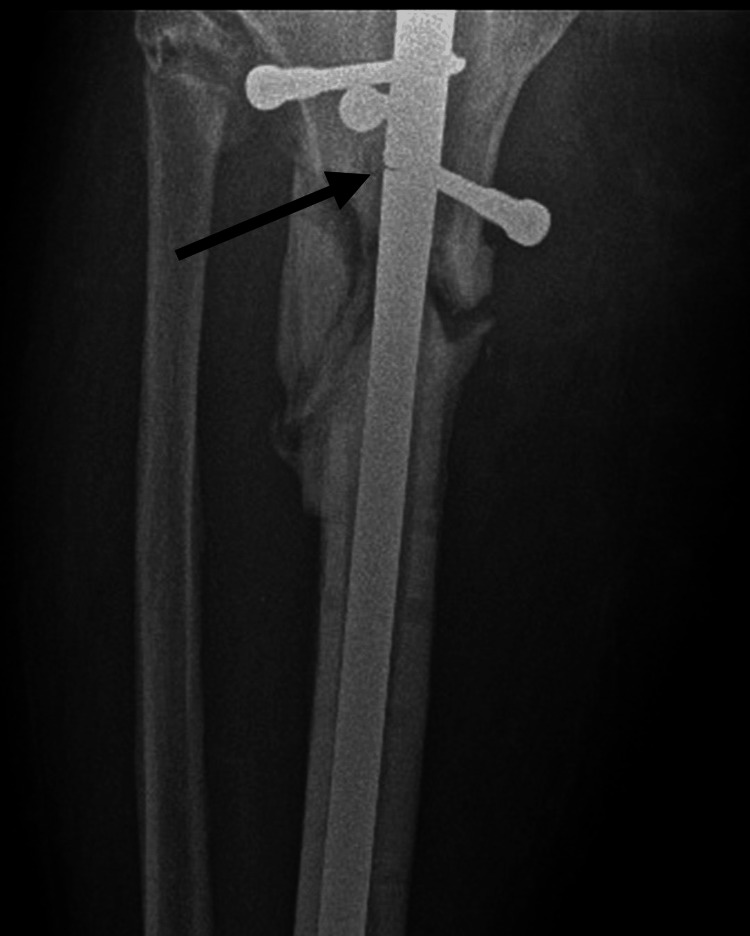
Anteroposterior X-ray seven months after the second surgical intervention, showing oligotrophic nonunion and implant failure (arrow points to the implant failure)
